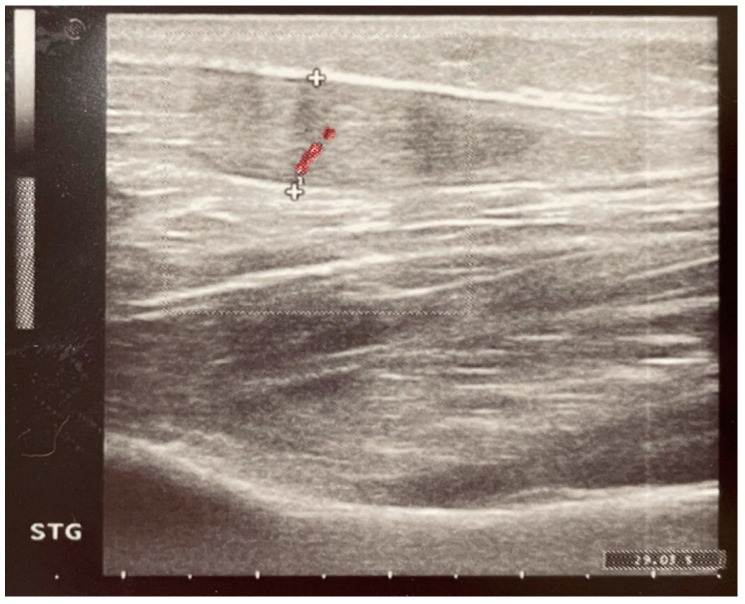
Longitudinal incidence ultrasound and color Doppler at 3 months showing an improvement in Achilles tendon thickness with decreased vascularization (red)—diameter of 6.6 mm.
